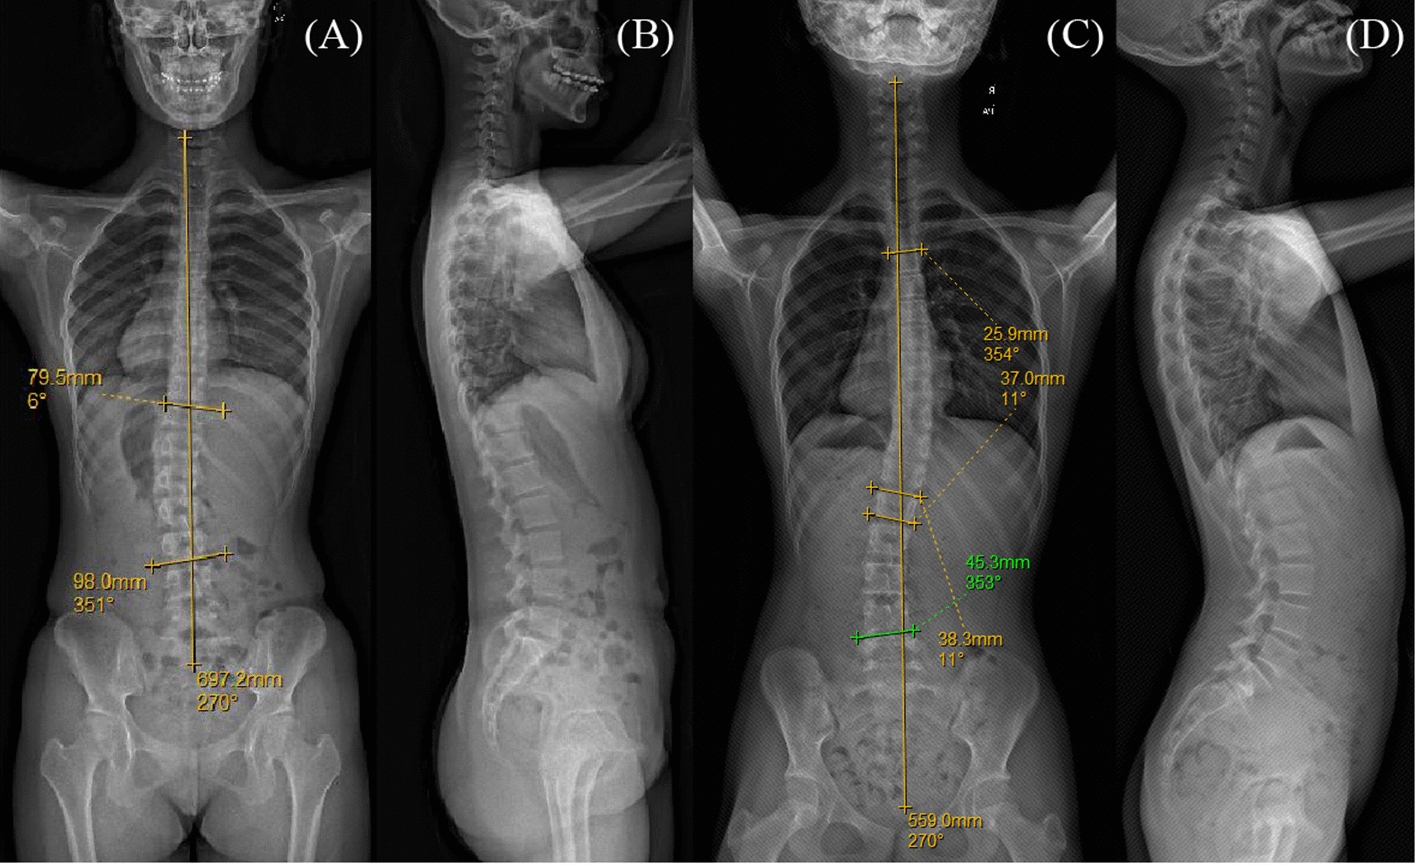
Frontal and lateral radiographic images of one PUMCI patient (A, B), frontal and lateral radiographic images of one PUMCII patient (C, D)
